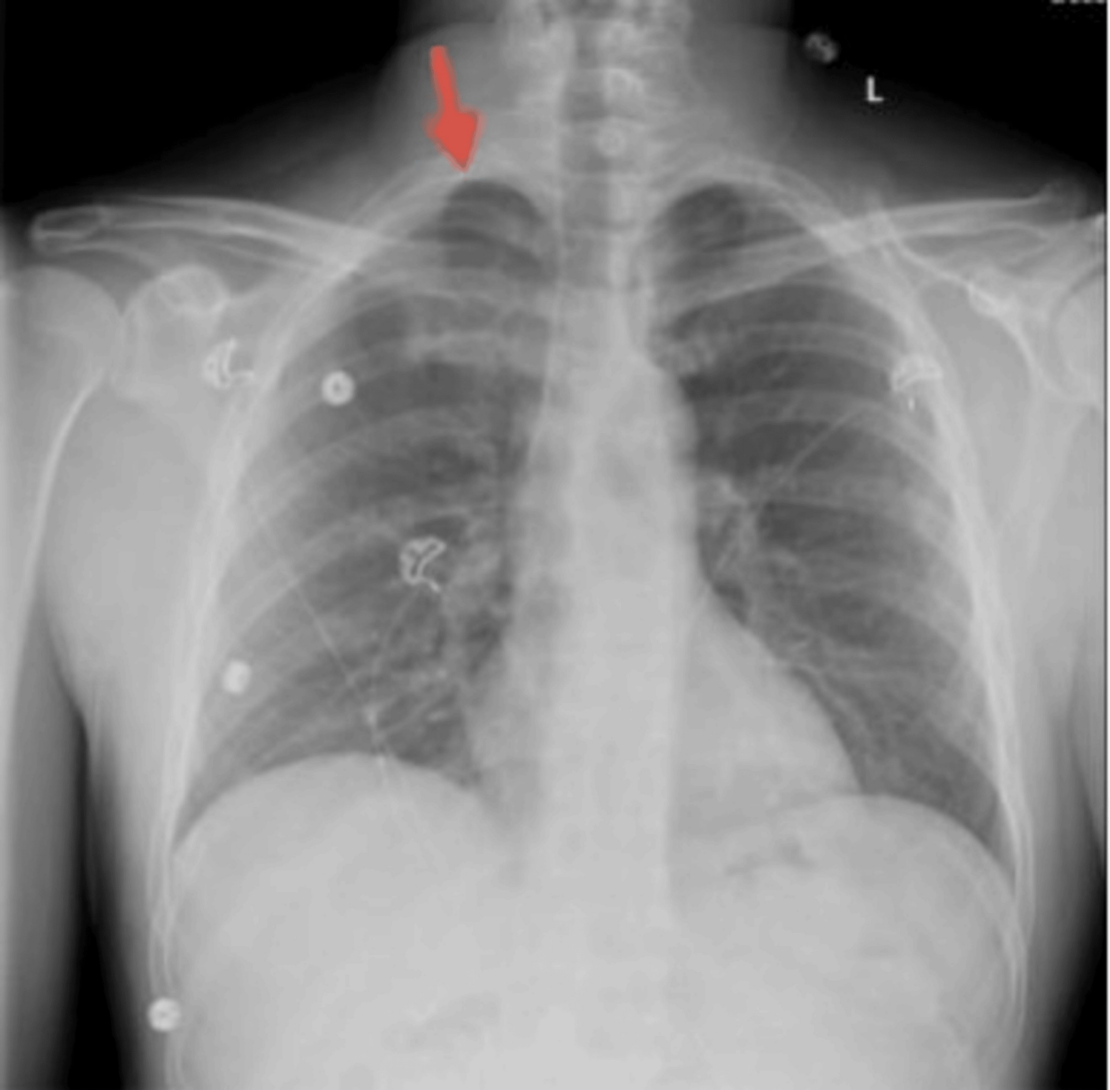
Chest X-ray with arrow pointing to the R apical pneumothorax (posteroanterior view)
